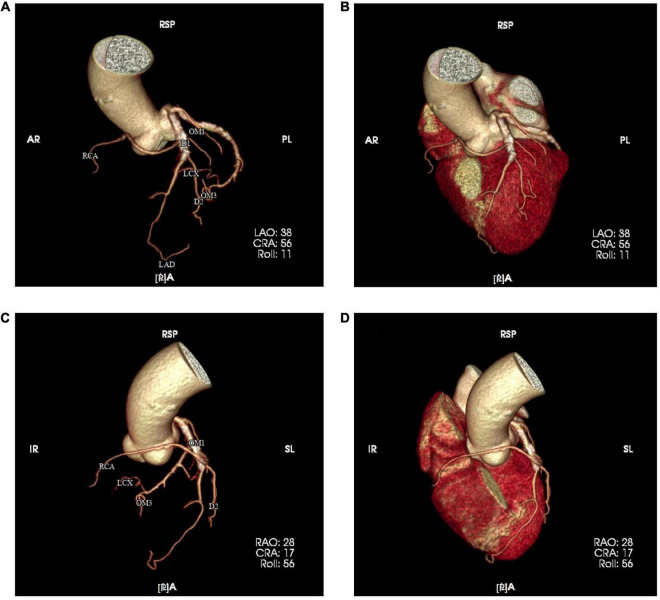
Coronary computed tomography angiography (CTA) was re-examined one month after PCI and resulting images (A–D) confirmed the anomalous origin of the right coronary artery.
